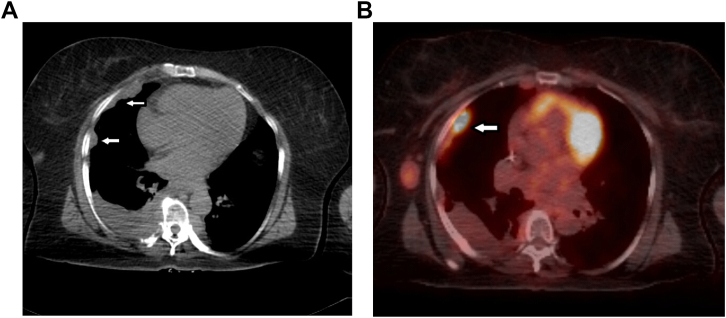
(A) Axial images chest CT scan which show soft tissue pleural based masses. (B) Axial images of PET/CT scan which show PET avid pleural based masses.
